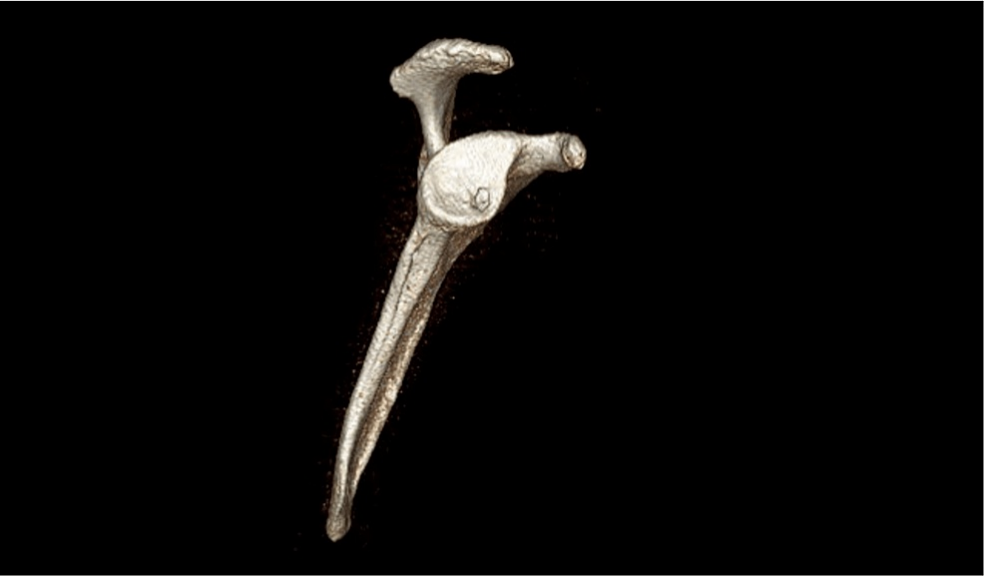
CT scan shows the location of the cyst.
